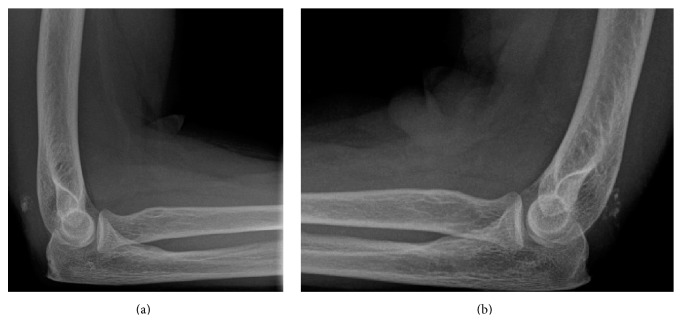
Lateral radiographs showing triceps tendon avulsion with a small piece of bone from the olecranon of the left (a) and right (b) elbow.
